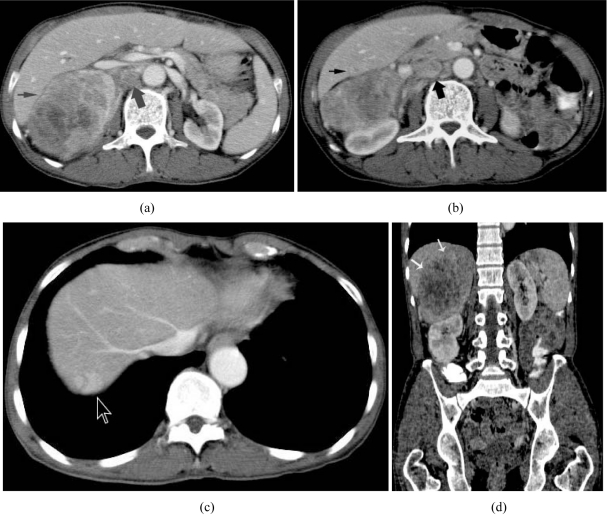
(a) Enhanced axial CT image shows a large inhomogeneous right adrenal mass (thin arrow), and an enlarged retroperitoneal lymph node (thick arrow). The inferior vena cava is patent; (b) Enhanced axial CT image obtained more distally shows a minimal inhomogeneously enhancing liver nodule (arrow), and multiple enlarged retroperitoneal lymph nodes (thick arrows); (c) Enhanced axial CT image obtained at the dome of the liver shows an enhancing liver nodule (arrow); (d) Enhanced coronal reconstructed CT image shows a large inhomogeneous right adrenal mass (arrows) with displacement of the right kidney downward.
